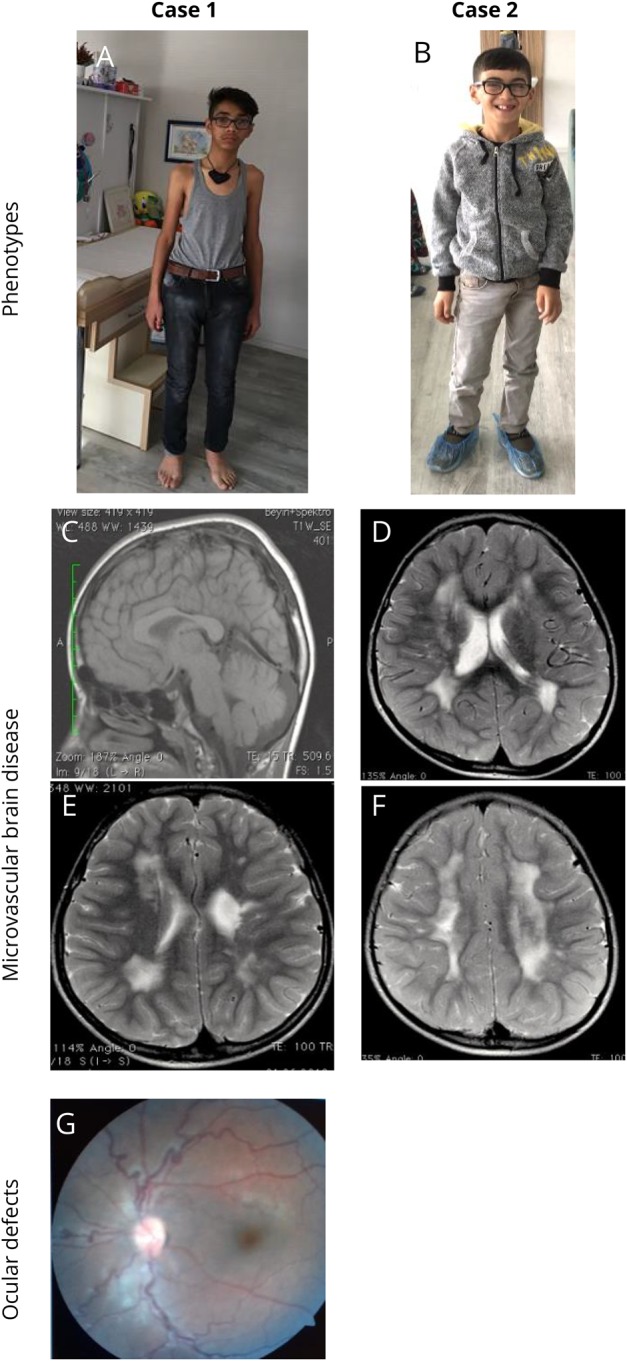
Selection of phenotypes, brain magnetic resonance images, and ocular defects of 2 cases (A) Case 1 (homozygous missense [p.Gly1278Ser] in *COL4A1*), aged 16 years at the last follow-up, had good academic skills with a marfanoid appearance, mild hemiparesis, partial seizures, and ocular defects. EEG was abnormal. Brain MRI findings showed that diffuse PVL with left ventricular enlargement with small porencephaly and thinning of the body part of the corpus callosum were stable even after 6 years. (B) Case 2 (homozygous missense [p.Gly1278Ser] in *COL4A1*), aged 10 years at the last follow-up, had good academic skills with mild hemiparesis and ocular defects. EEG was normal. Brain MRI findings showed that PVL with right mild ventricular enlargement was stable even 6 years later. (C) T1-weighted sagittal image of case 1, showing thinning of the body part of the corpus callosum. (D) T2-weighted axial image of case 2, showing diffuse PVL with right mild ventricular enlargement. (E) T2-weighted axial image of cases 1, showing diffuse PVL with left ventricular enlargement and small porencephalic cyst. (F) T2-weighted axial image of cases 2, showing diffuse bilateral deep white matter leukoencephalopathy. (G) Ophthalmoscopic examination of case 1 showing hypoplastic optic discs and tortuosity of retinal veins. PVL = periventricular leukoencephalopathy.
